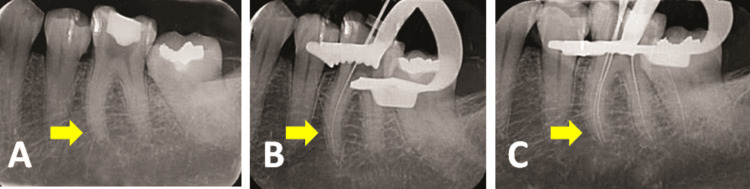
Intraoral periapical radiographs of 36 (A) A 15 K file separated in the apical third of the mesiobuccal canal, (B) separated file bypassed using a 15 K file, and (C) working length determination
